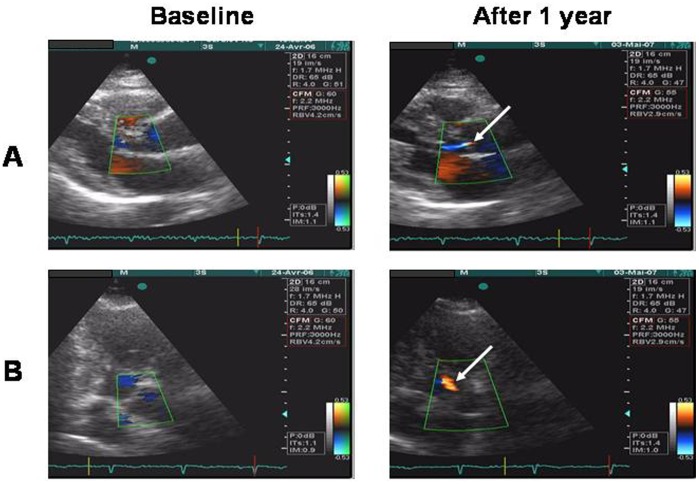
Example of an emergent grade 1 aortic regurgitation in a patient treated with benfluorex. In the parasternal views (row A) and the apical 3-chamber views (row B), grade 1 aortic regurgitation is shown by colour Doppler (arrow) after 1 year of treatment with benfluorex.
